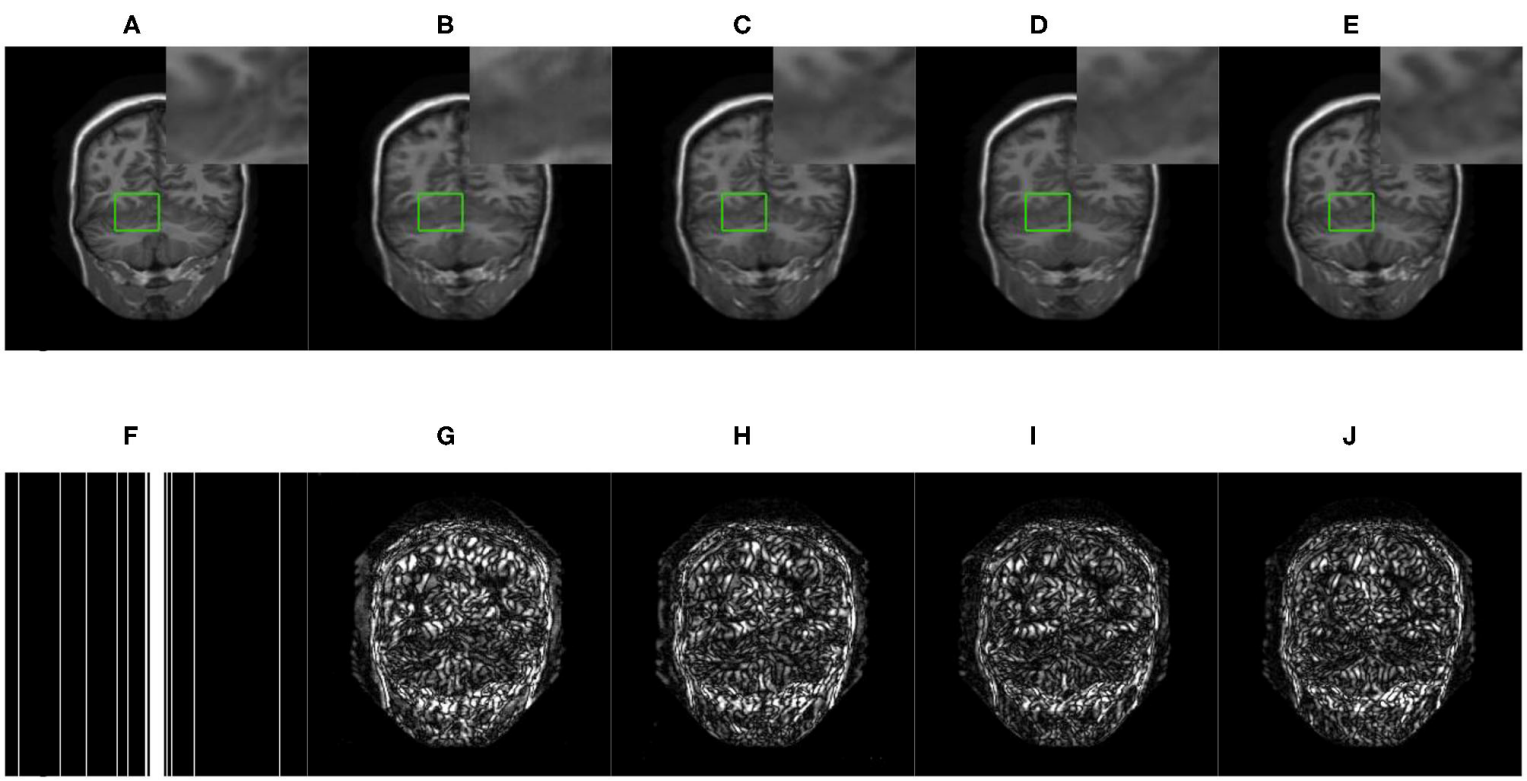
The reconstructed MRI for the 10-fold accelerated k-space data masked with the 1D Gaussian distribution by using different GAN-based methods. (A) Fully-sampled MRI, (B) DAGAN, (C) DAWGAN, (D) DAWGAN-GP, (E) SARA-GAN, (F) 1D mask, (G) DAGAN(error), (H) DAWGAN(error), (I) DAWGAN-GP(error), and (J) SARA-GAN(error).
